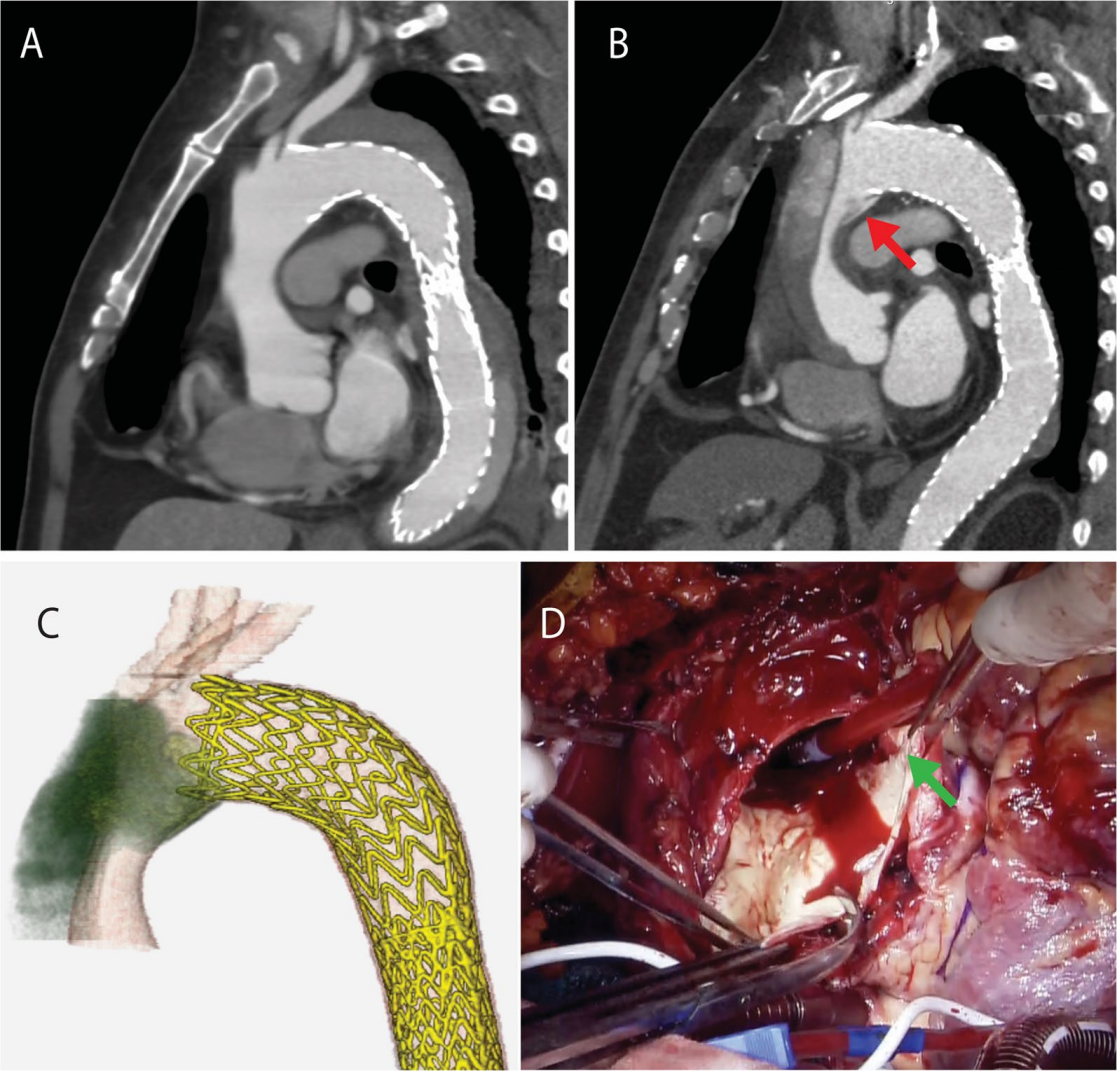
Preoperative and intraoperative images. A Computed tomography after thoracic endovascular aortic repair 7 years previously. B, C Preoperative computed tomography showed proximal stent graft-induced new entry at the aortic arch (red arrow). D Intraoperative photograph showed entry (green arrow)
